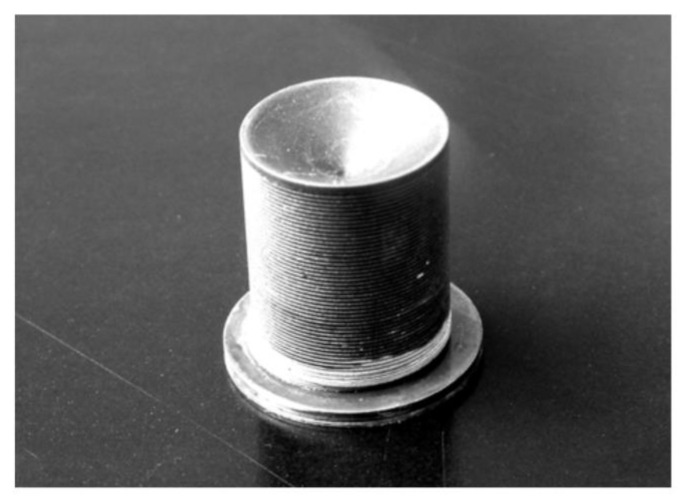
High temperature ultrasonic transducer with an integral concaved backing.
